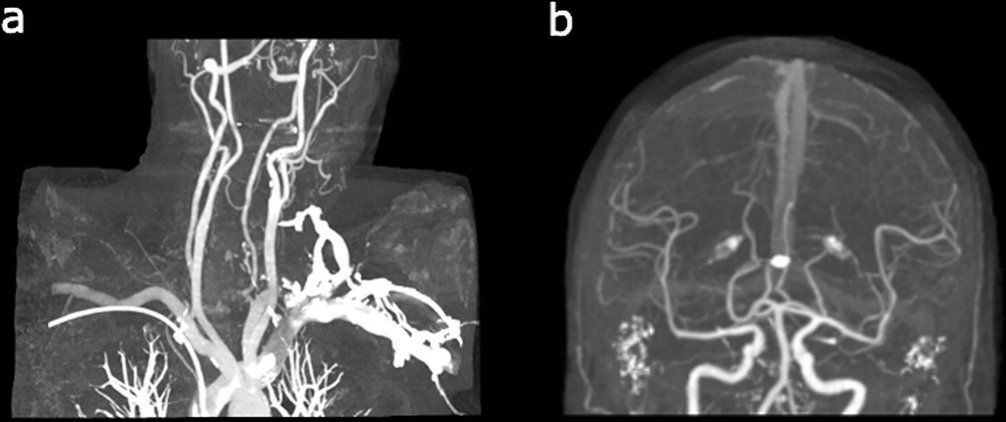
The patient’s CT angiogram of (a) the carotids showing no significant stenosis and (b) the intracranial blood vessels.
